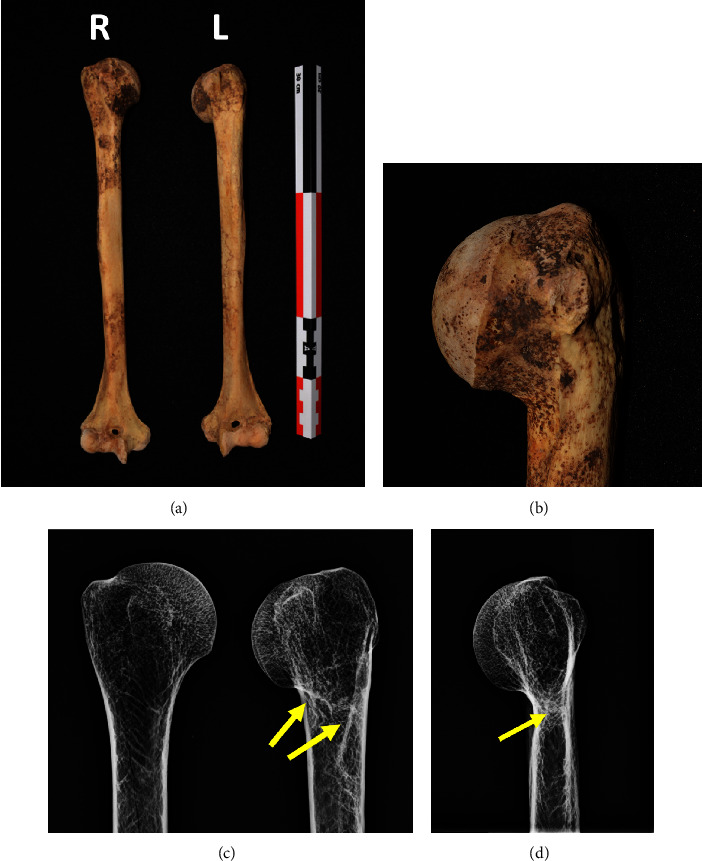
A well-healed fracture of the left humerus surgical neck (This is an original figure created by the authors of the manuscript). (a) An anteroposterior photograph of both the right and left humerii. Note the shorter left humerus; the left humerus was 309 mm in length and the right one was 323 mm in length. (b) A close-up photograph of the left proximal humerus demonstrating a varus neck shaft position with inferior displacement of the humeral head. (c) An anteroposterior radiograph of both proximal humerii. Note the internal callus of the left humerus denoting a well-healed longstanding fracture (yellow arrow). (d) A lateral radiograph of the left proximal humerus demonstrating the internal callus (yellow arrow) and distorted surgical neck. Malunions such as this are common in fractures of the humeral neck treated nonoperatively [22].
